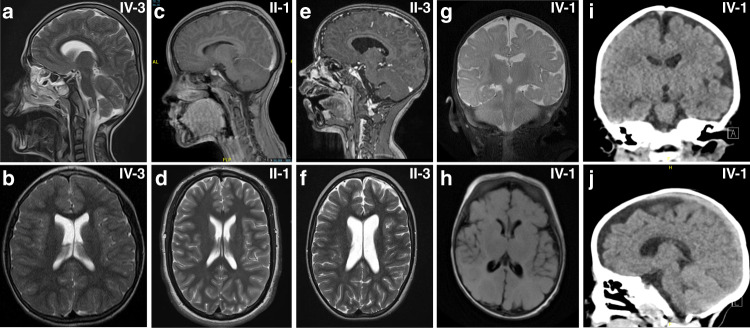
Neuroradiologic features of affected individuals. Sagittal (a) and axial (b) T2-weighted images of subject IV-3 from family 1 at the age of 10 revealed no structural brain anomalies but showed microcephaly and thin cerebral cortex. (c) Sagittal T1 section after gadolinium injection of subject II-1 (family 4) at 10 years of age and (d) axial T2-weighted images at 16 years revealed no brain-specific abnormality except for a discreet prominent aspect of the lateral ventricles. (e) Sagittal T1 section after gadolinium injection and (f) axial T2-weighted images of subject II-3 (family 4) at 7 years of age revealed a prominent aspect of the lateral ventricles, of the 3rd and, to a milder degree, of the 4th ventricle. (g) Coronal T2-weighted and (h) axial T2-Flair images of subject IV-2 (family 6) at 3 months of age showing normal signal intensity, age-appropriate myelination process and slightly enlarged cerebrospinal fluid (CSF). Coronal (i) and sagittal (j) computed tomography (CT) images of the same subject at the age of 3 years revealing left-sided subcortical hypodensity within left temporal lobe and confirming prominent CSF space.
